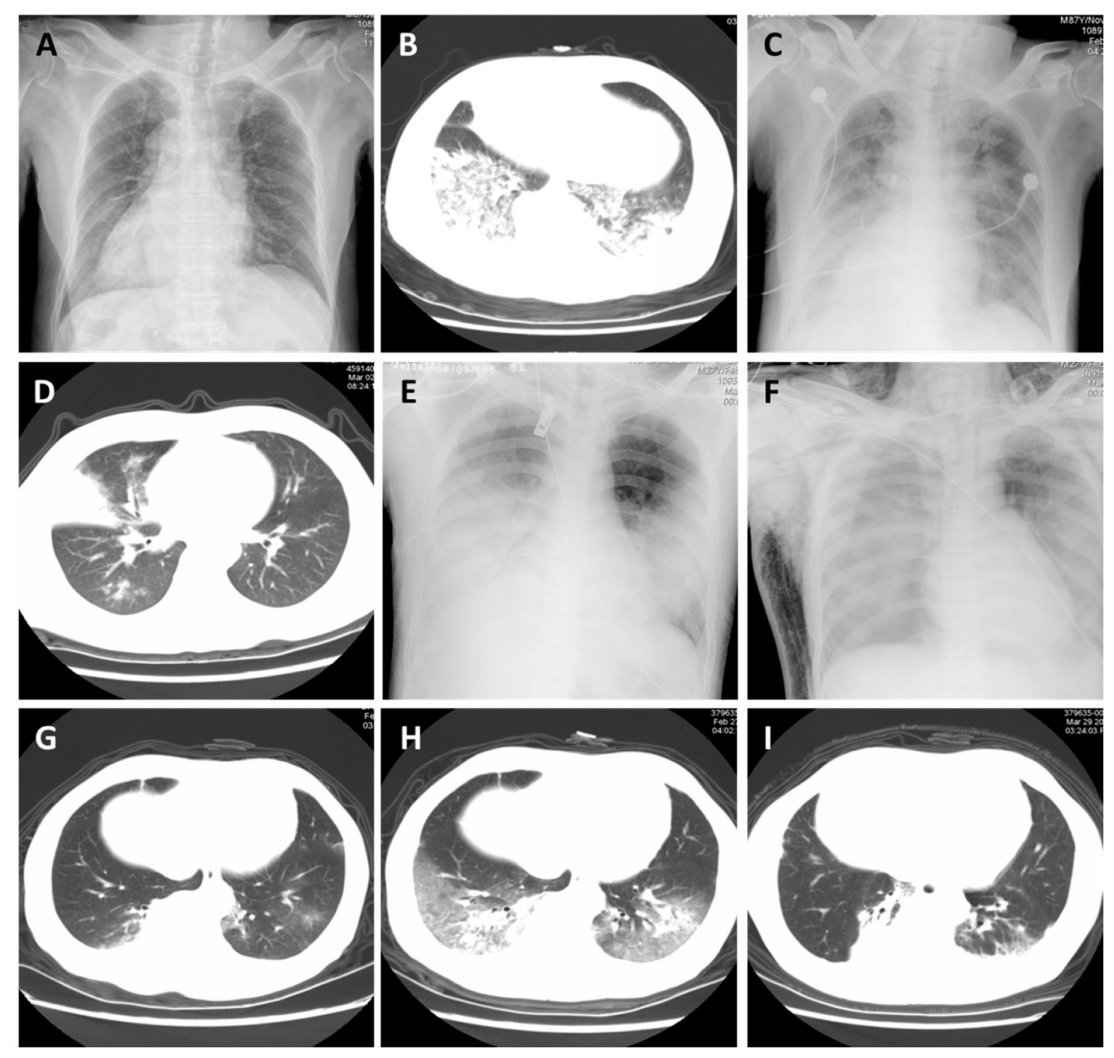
Chest radiographic findings and progression of pneumonia in 6 patients with H7N9 infection. Case 1: Panels A, B, and C; Case 4: Panels D, E, and F; Case 2: Panels G, H, and I. Early stage (panels A, D, and G): Chest computed tomography and radiograph show progressive consolidation in the lungs; Progressing stage (panels B, E, and H): Chest computed tomography and radiograph show bilateral ground-glass opacity and consolidation; Final stage (panels C, F, and I): Chest computed tomography and radiograph show diffuse lesions and bilateral involvement of the lungs (white lungs) in panels C and F, subcutaneous emphysema with pneumomediastinum in panel F, and diffuse lesions are obviously seen in panel I.
